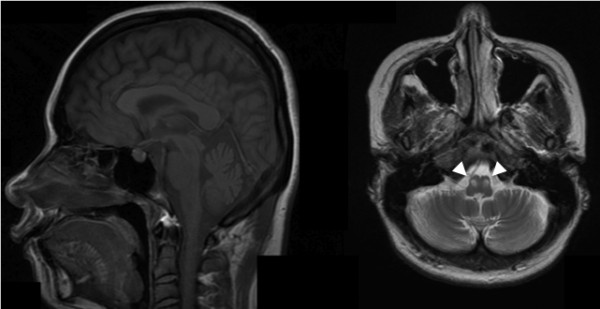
Brain MRI. Left is T1 weighted sagittal image, and right is T2 weighted axial image. Cerebellar atrophy was observed. Bilateral inferior olives showed T2 high intensity (arrowheads).
